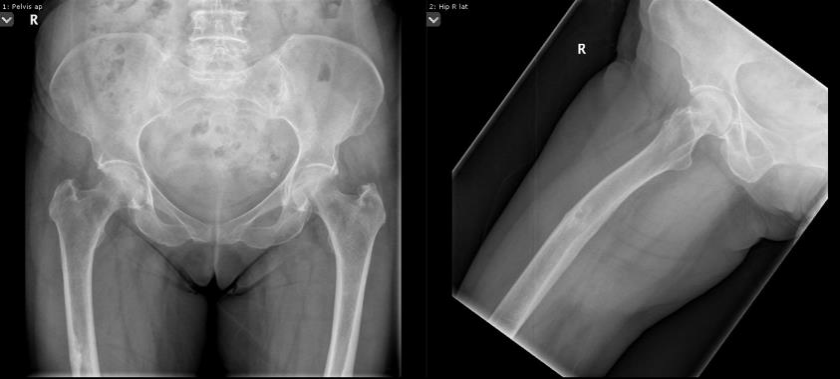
Radiograph Showing Incomplete Atypical Femoral Fracture of the Right Femur with Lateral Cortical Thickening.
